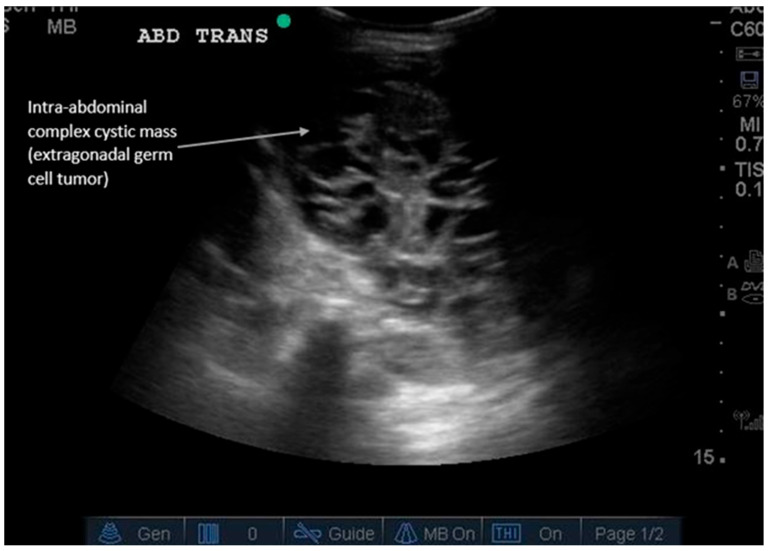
Incidental abdominal mass. Abdominal masses can present differently depending on their internal composition. Ultrasound is the first-line modality for palpable masses because it can be performed at the site of palpation, determine echogenicity, and guide biopsy for definitive diagnosis. Supplementary Video S6 shows dynamic findings of an extragonadal germ cell tumor presenting as a complex, cystic intra-abdominal mass in an infant. Masses can be solid (hyperechoic), cystic (anechoic), or fatty (e.g., lipomas); simple or complex (can have septations); or can contain calcifications (1–2 mm small hyperechoic structures with posterior shadowing). Adding color Doppler can evaluate vascular flow within the mass (positive flow is more suggestive of malignancy). A round vs. irregular shape also helps determine whether it is benign or malignant, respectively. Ultrasound is particularly useful in pediatric abdominal masses to avoid radiation from CT scans and allow expedited diagnosis. Types of pediatric malignant masses include Wilms’ tumor (typically intrarenal), hepatoblastoma (liver), neuroblastoma, non-Hodgkin’s lymphoma, germ cell tumor (ovarian or testicular), and others [27]. Subsequent imaging for detected masses includes Magnetic Resonance Imaging (MRI) for indeterminate fat-containing or solid masses and CT for fluid collections after trauma or surgery or for suspected metastases requiring restaging imaging [18,27]. Supplemental Table S1 summarizes the major diagnostic findings for each GI pathology described and the recommended locations for POCUS scanning. Supplemental Table S2 describes the sensitivity and specificity of ultrasound compared to computed tomography (CT) for various gastrointestinal pathologies [13,14,15,18,19,20,21,24,26,27,28]. Conclusions: In summary, POCUS is a validated, guideline-endorsed tool for the rapid initial assessment of common GI pathologies. Its integration into clinical pathways for abdominal pain can improve diagnostic efficiency, reduce radiation exposure, and expedite definitive care.
